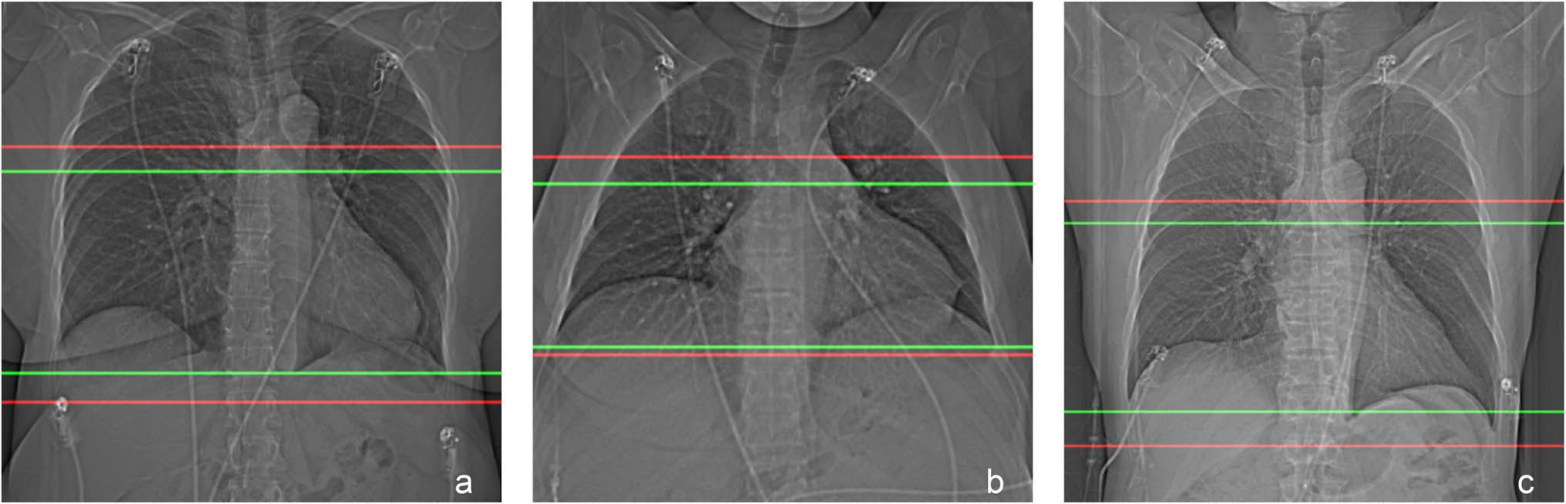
CT localizers for three patients with a scan range from the radiologists. The radiologists’ scan range is depicted in green, and the radiographers in red. a The CT localizer of a female patient (55 years). b The CT localizer of a male patient (36 years). c The CT localizer of a female patient (59 years)
